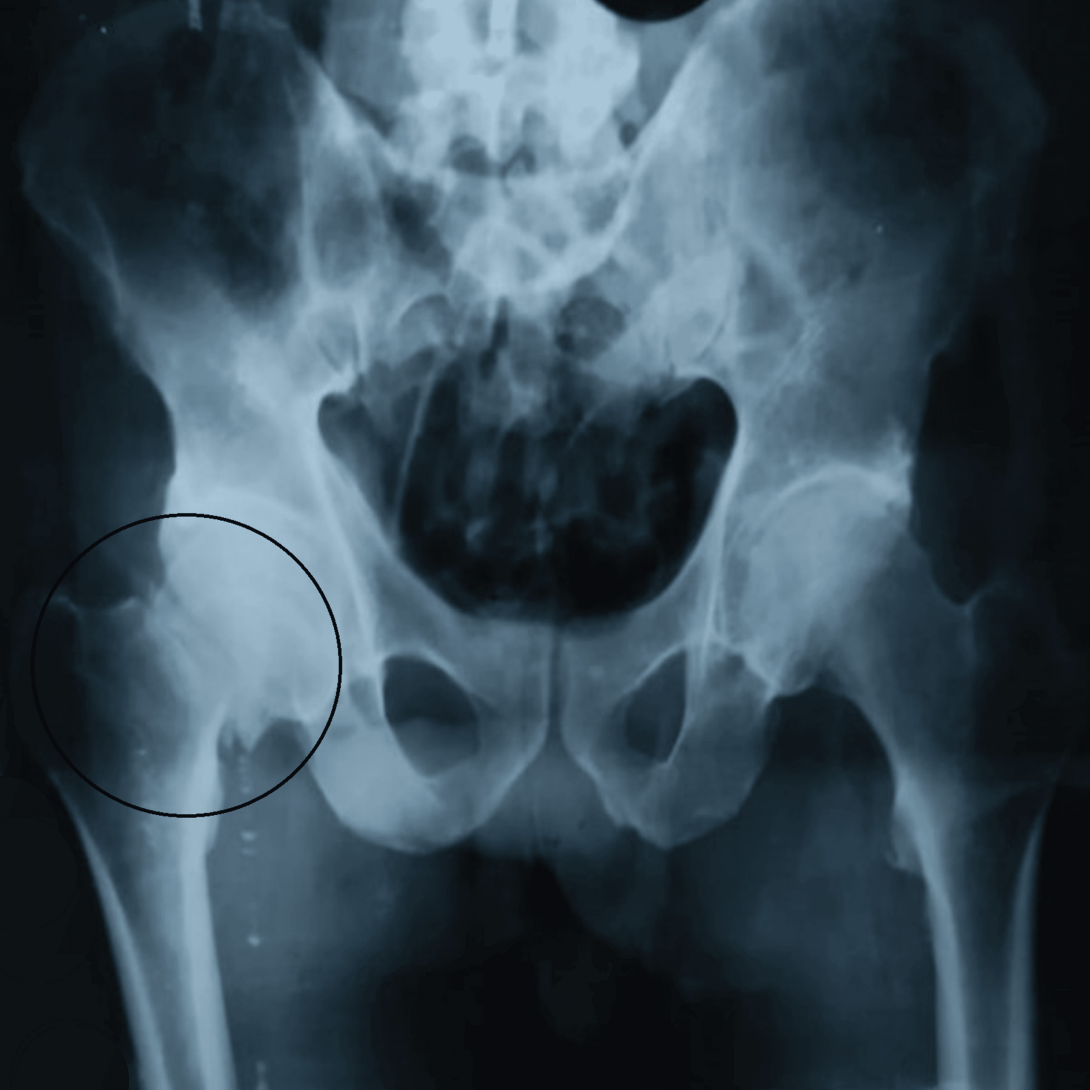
X-ray of pelvis with both hips (anteroposterior) showing right-sided femoral neck fracture (Garden Type IV and Pauwels Type III)
